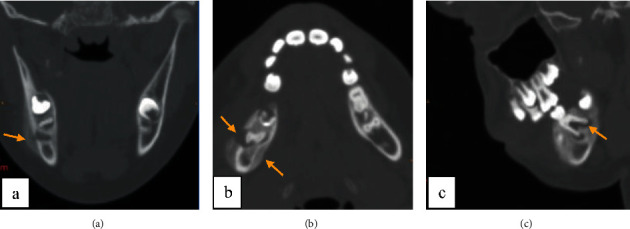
Computed tomography exam: (a) Coronal section showing “onion skin” aspect of lateral periostitis and the radiolucency in relation with first molar's roots. (b) Axial section showing periostitis in both external and internal mandibular cortex with lateral rupture of the cortical. (c) Sagittal section showing radiolucency in relation with first molar's roots.
